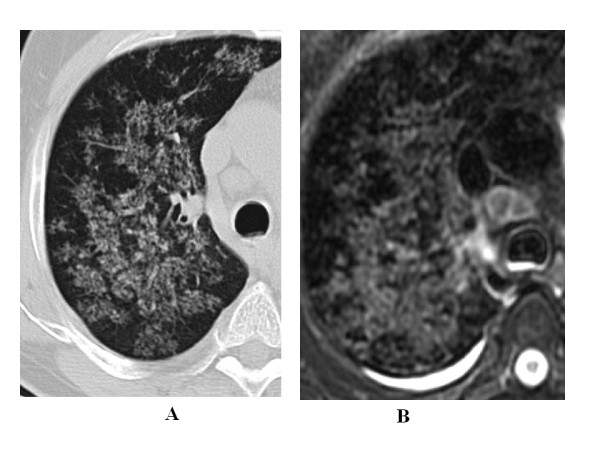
53 year-old woman with pulmonary tuberculosis. A) HRCT shows interstitial changes with peribronchiolar thickening. B) Unenhanced MRI identifies the same features as well as HRCT and also depicts lymph node caseosis.
